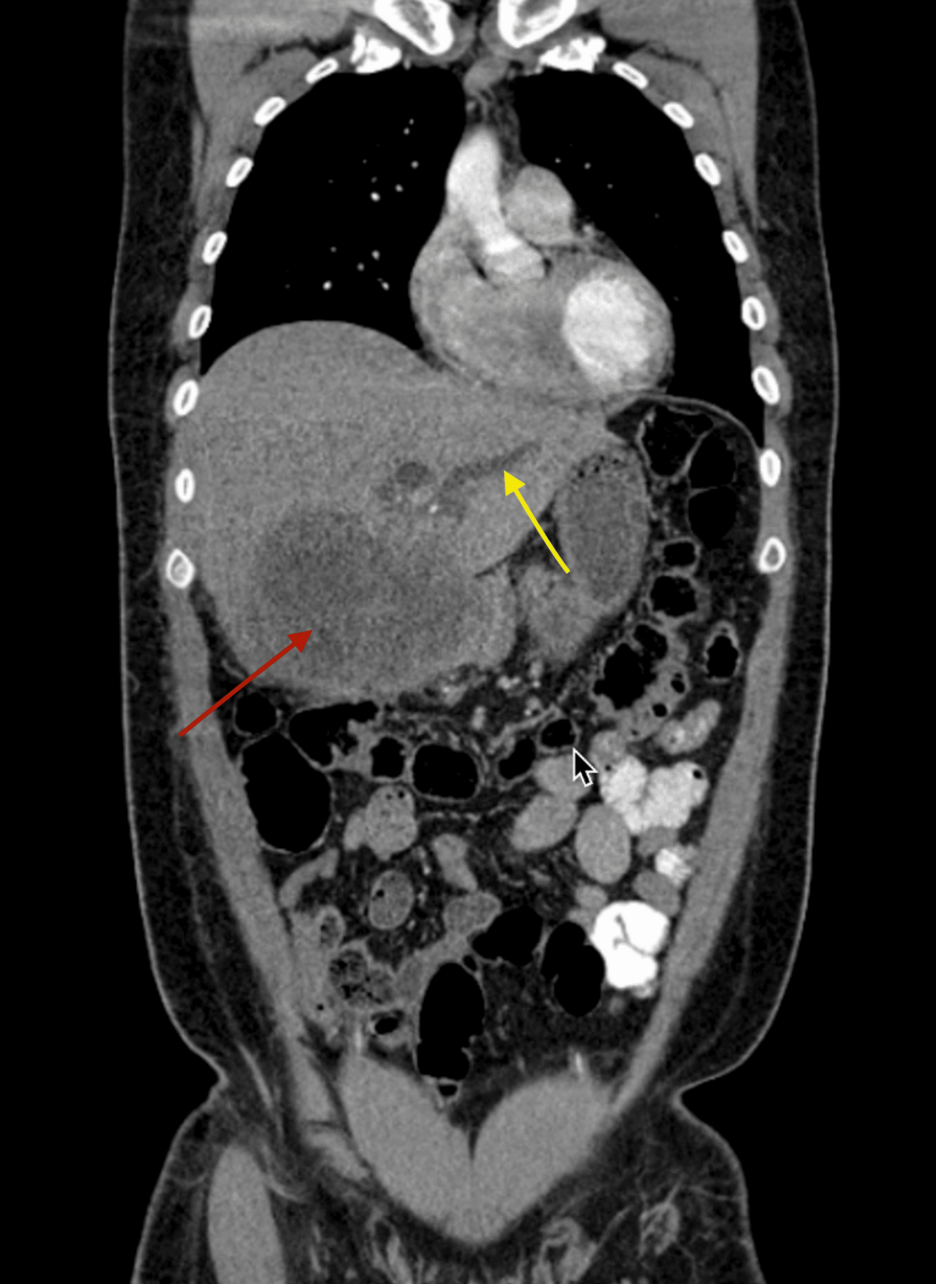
Contrast-enhanced computed tomography of the chest and abdomen In the coronal sections, the intrahepatic mass with ill-defined borders (red arrow), causing marked dilation of the intrahepatic bile ducts (yellow arrow)
